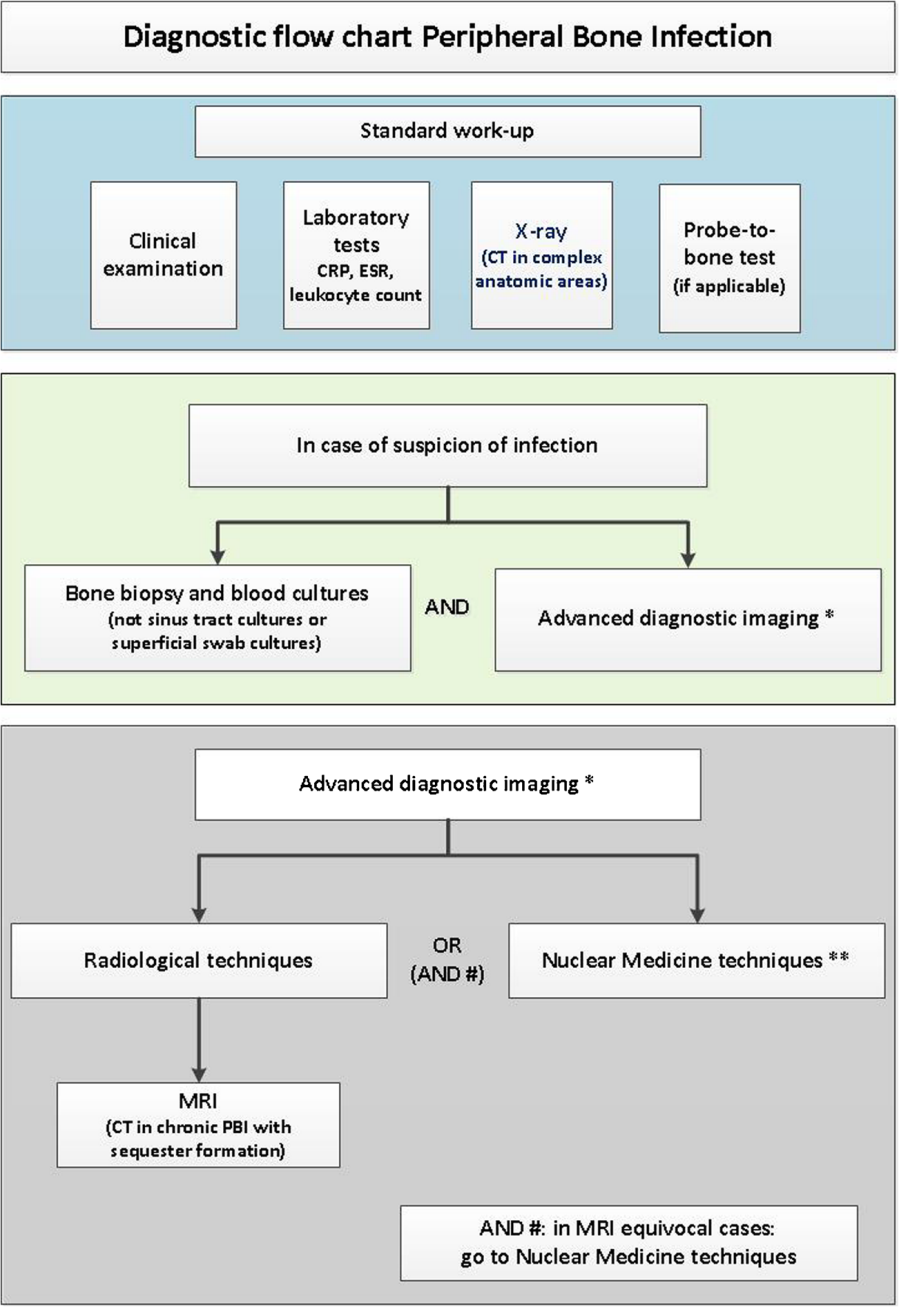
The suggested initial diagnostic steps to undertake in the suspicion of PBI, based on published evidence. Of course not all steps are required, this may change in the individual patient. Some steps can of course be repeated when necessary. Serological tests can be performed over time since the trend to increase or decrease is more important than a single value. At this moment, there is not enough clinical evidence to support the use of one advanced diagnostic imaging technique above the other. There is a lack of studies with large patient numbers and there are hardly no comparative studies. Therefore, the choice of which advanced diagnostic modality to be used first depends on several factors, such as experience of the imaging specialist, costs, availability, radiation burden and local expertise (see also Tables 1 and 2). In many hospitals, MRI is considered as the first advanced imaging modality in daily practice, mainly because there is no radiation involved. In patients with metallic hardware, however, there is sufficient literature to support a preferential use of white blood cell scintigraphy
